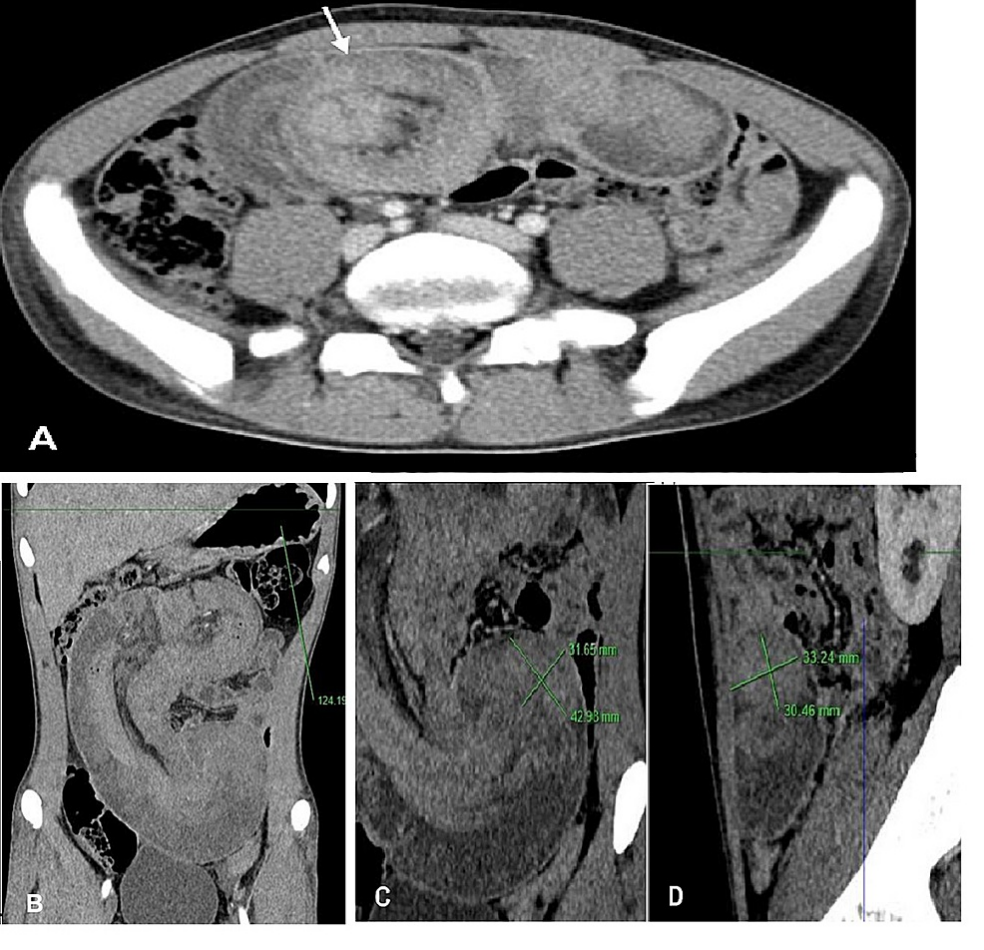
Enteroenteric intussusception presents a round mass as a “target” pattern on axial CT image (A) and as a large “sausage-shaped” pattern on coronal CT image (B), with a central hypodense area of mesenteric fat (arrow). Oblique (C) and sagittal (D) CT images depict a lead point of the mass at the apex of the jejunal intussusception.
